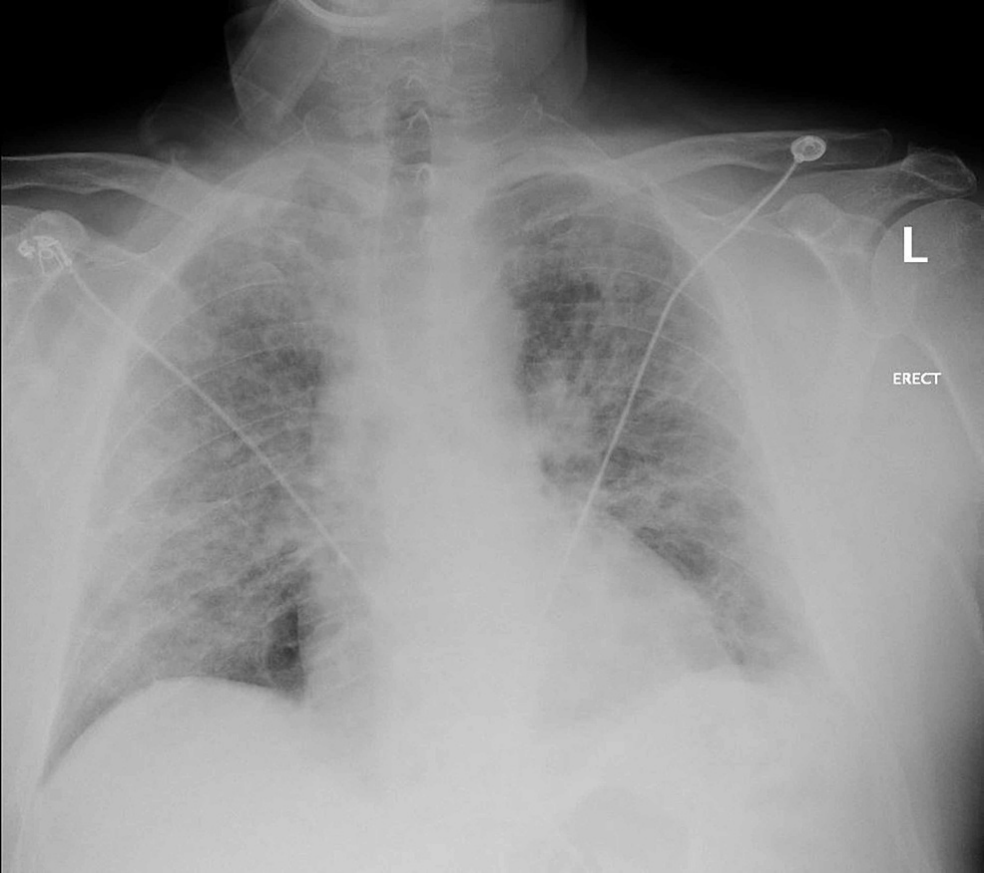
Chest X-ray on presentation showing diffuse patchy/interstitial opacities consistent with atypical infection No frank lobar consolidation is seen.
